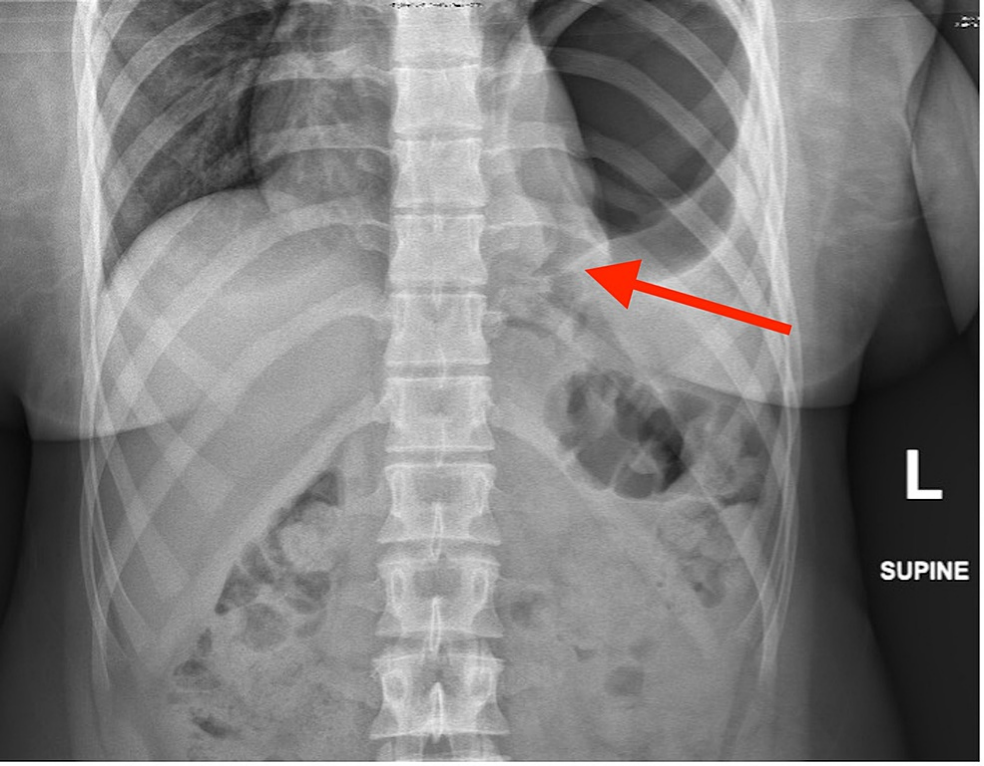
X-ray showing bowel passing through the diaphragmatic defect into thoracic cavity
